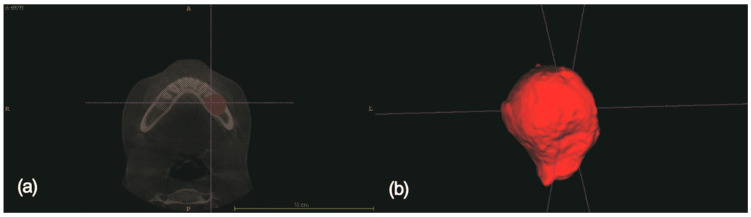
(a) Segmentation of the cystic lesion in axial plane of computed tomographic data. (b) Computerized three-dimensional reconstruction of the cystic lesion. Volume was measured with ITK-SNAP (Penn Image Computing and Science Laboratory, PA, USA).
